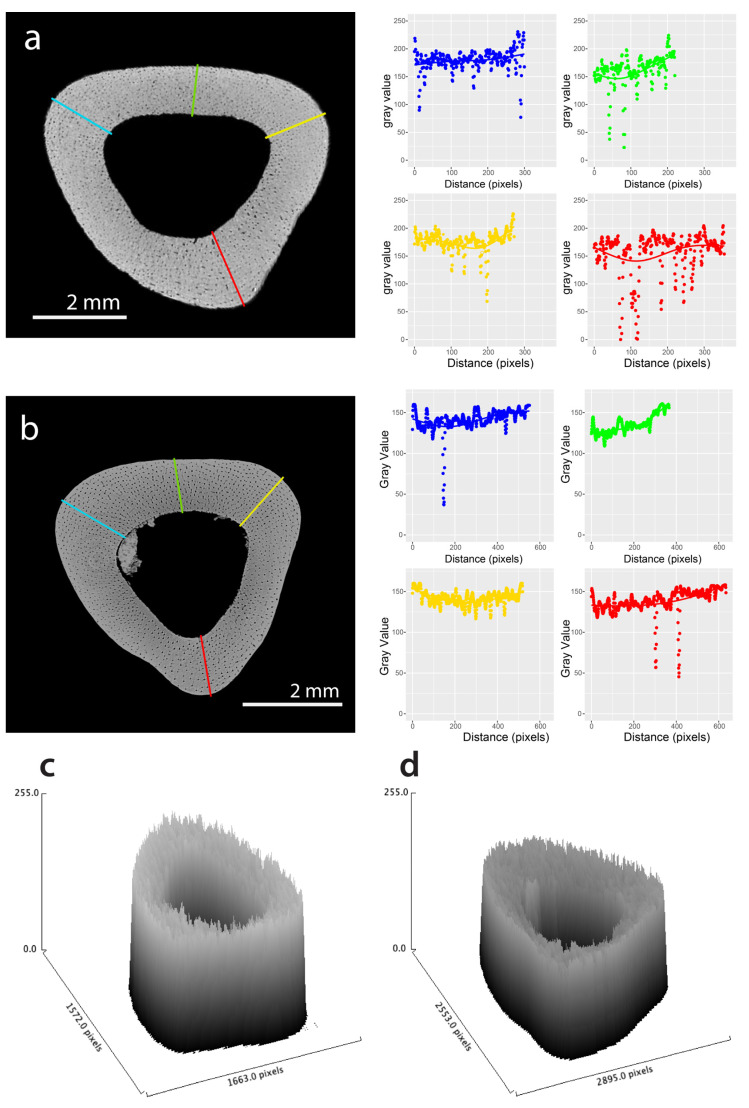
Bone density profiles of perinate tyrannosaurids. Bone density profiles for (a) *T. rex* RSKM P2416.82; (b) *G. libratus* TMP 1981.16.475, using gray value as a proxy for density; surface maps of CT slice data for (c) *T. rex* RSKM P2416.82; (d) *G. libratus* TMP 1981.16.475.
